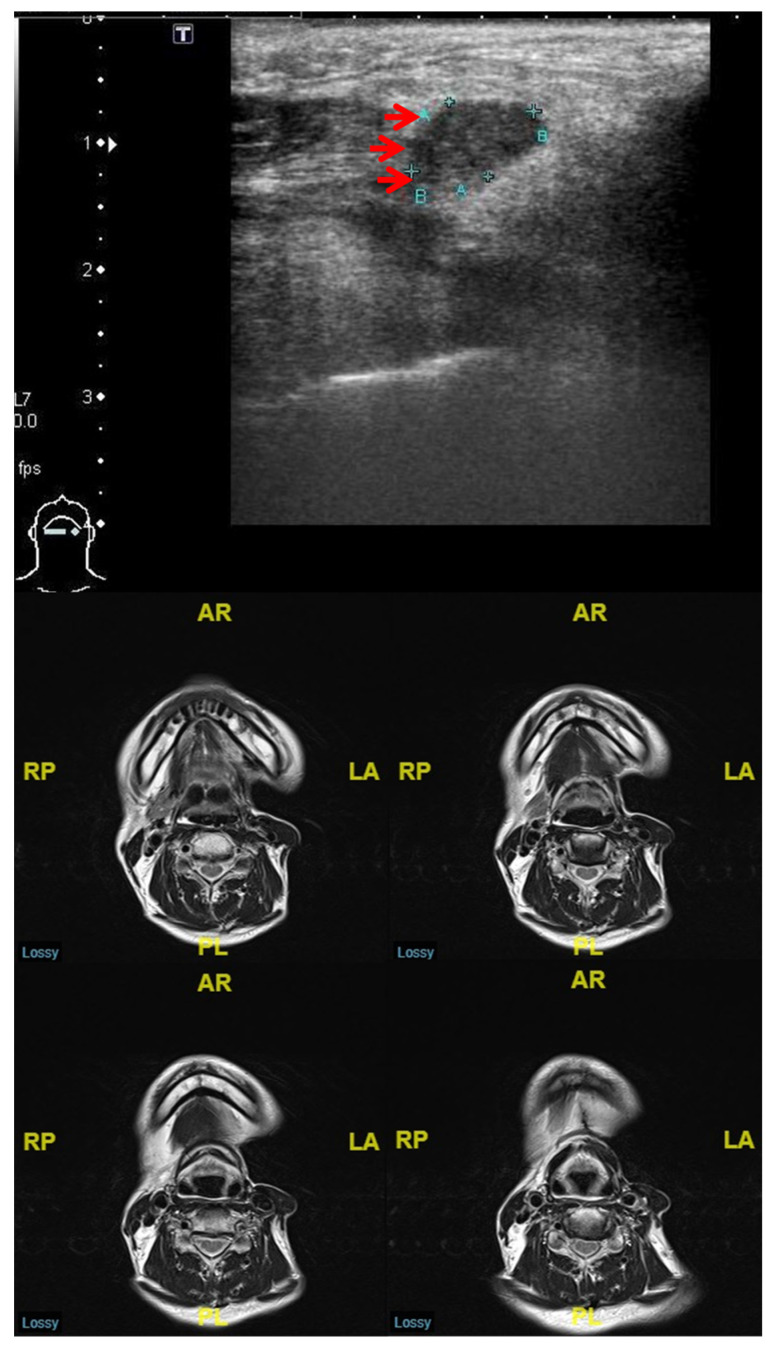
This is a 51-year-old male who was diagnosed with left tongue cancer status post-wide excision, left neck dissection, and adjuvant CRT. The 5-month post-treatment MRI reported no abnormal cervical LN (lower part of image). The US examination revealed a hypoechogenic mass (0.66 × 1.07 cm) over the right neck level IA (arrow, upper part of image). Both the cytological and pathological results demonstrated a metastatic LN.
